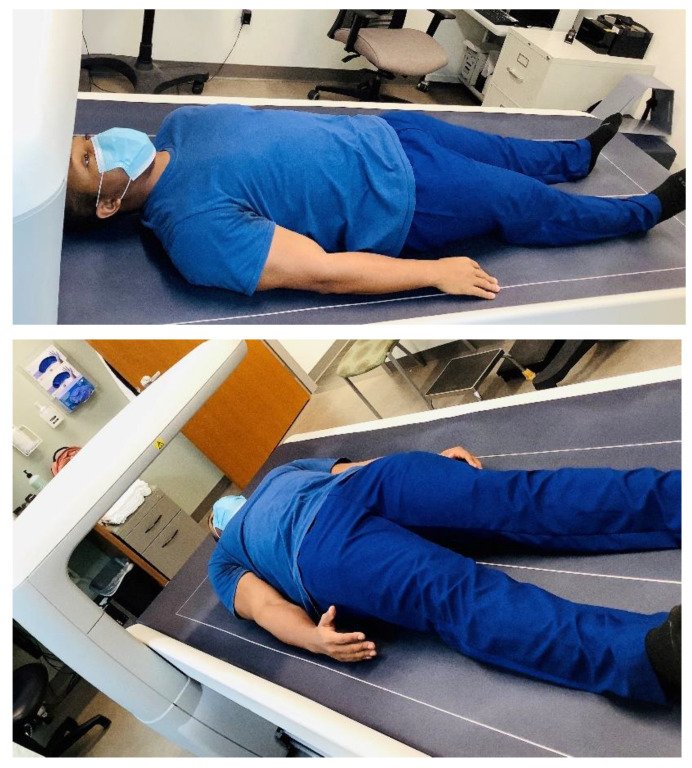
Dual-energy X-ray absorptiometry; photo courtesy of Clifton J. Holmes, PhD, in conjunction with the Clinical Translational Research Unit at the Washington University School of Medicine in St. Louis.
